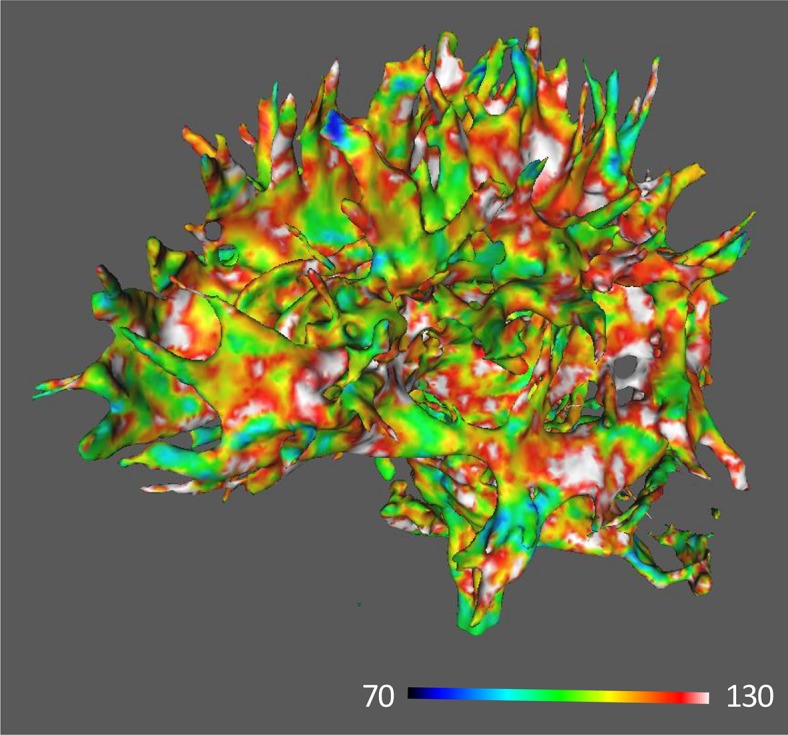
MRI image of the highest FA-BHQ score.
